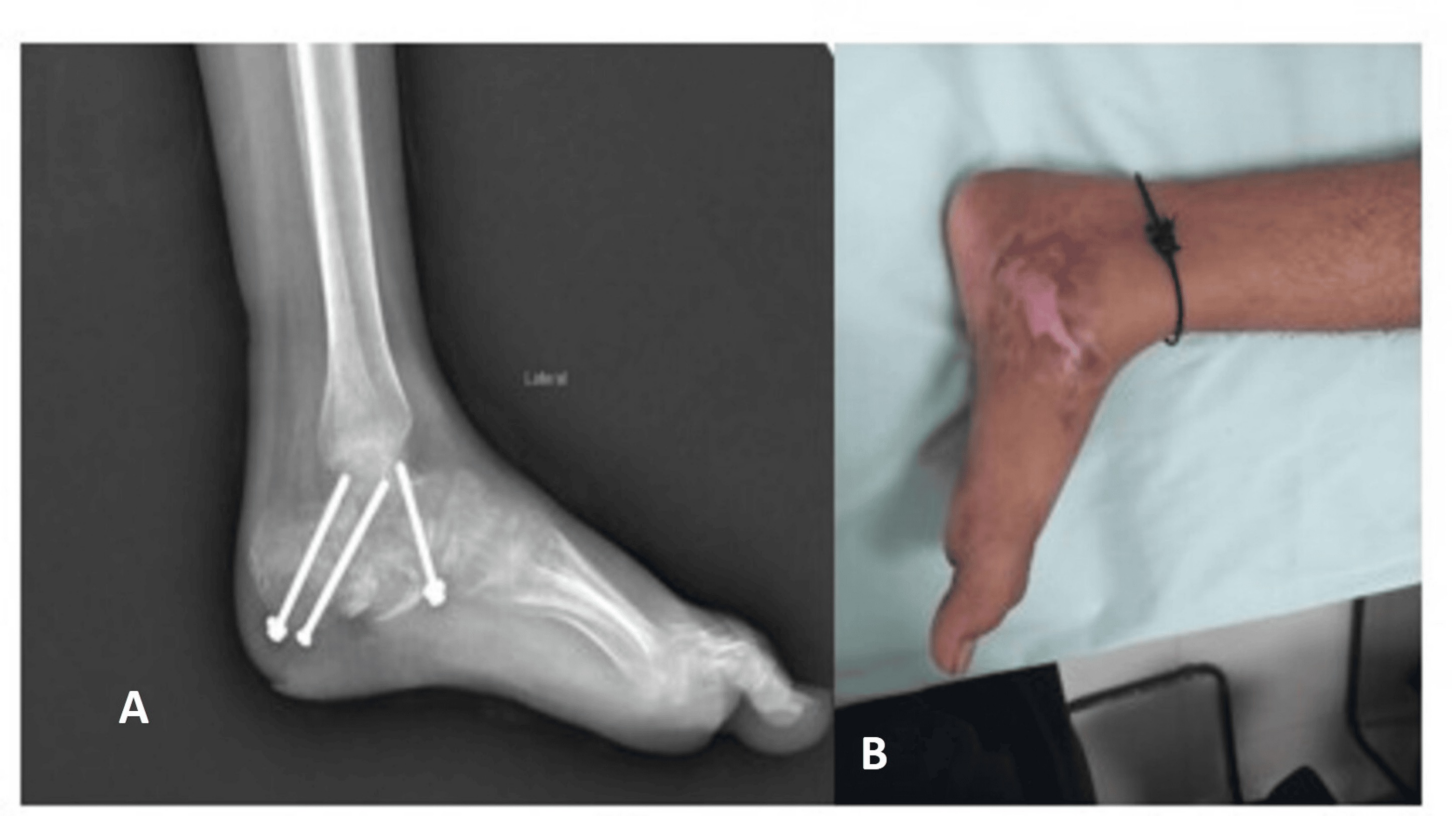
Follow-up radiograph and healed scar. (A) Anteroposterior and lateral three-month follow-up radiograph of the foot showing screws in position and evidence of healing. (B) Wound healed by secondary intention by granulation tissue.
